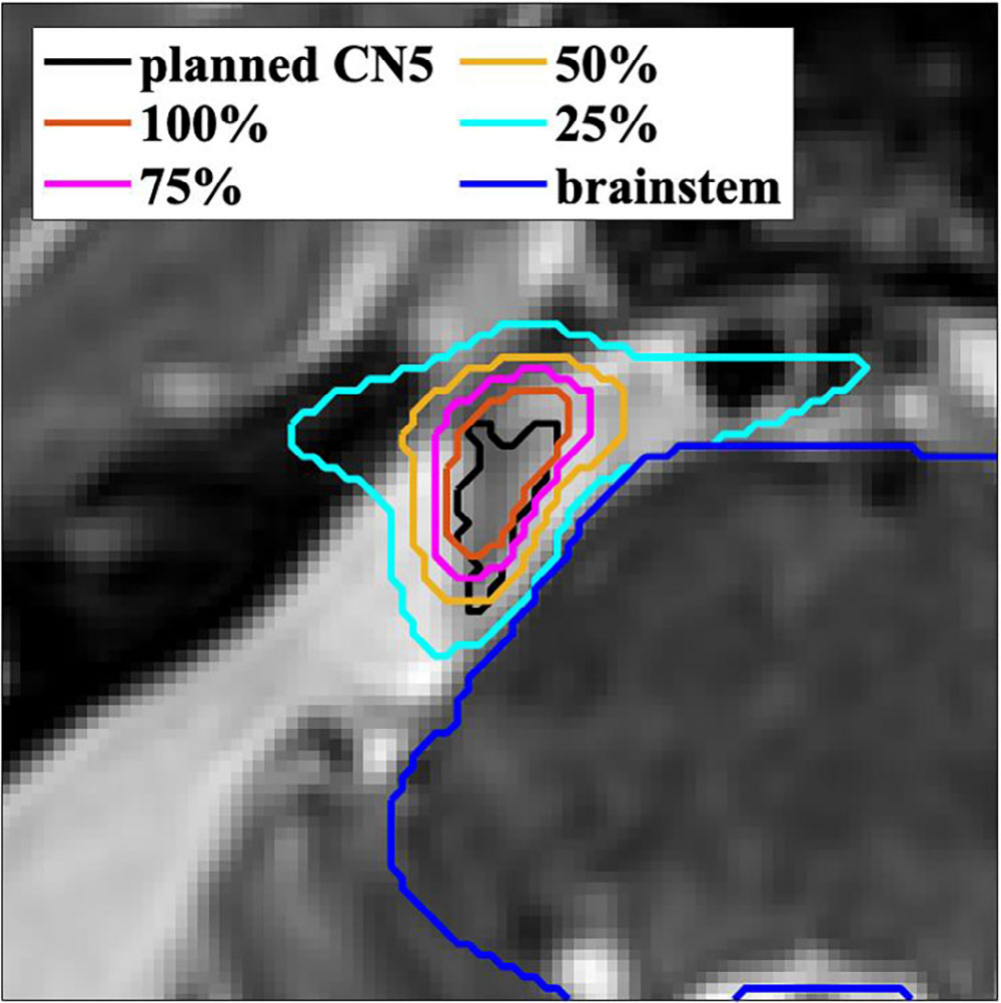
The isodose lines and contours of the trigeminal nerve (CN5) and the brainstem are shown on a SPACE T2‐weighted MR image (transverse plane). The pixel size is 0.5 mm × 0.5 mm, and the dose falls from 100% to 25% of the prescription dose within a few millimeters.
